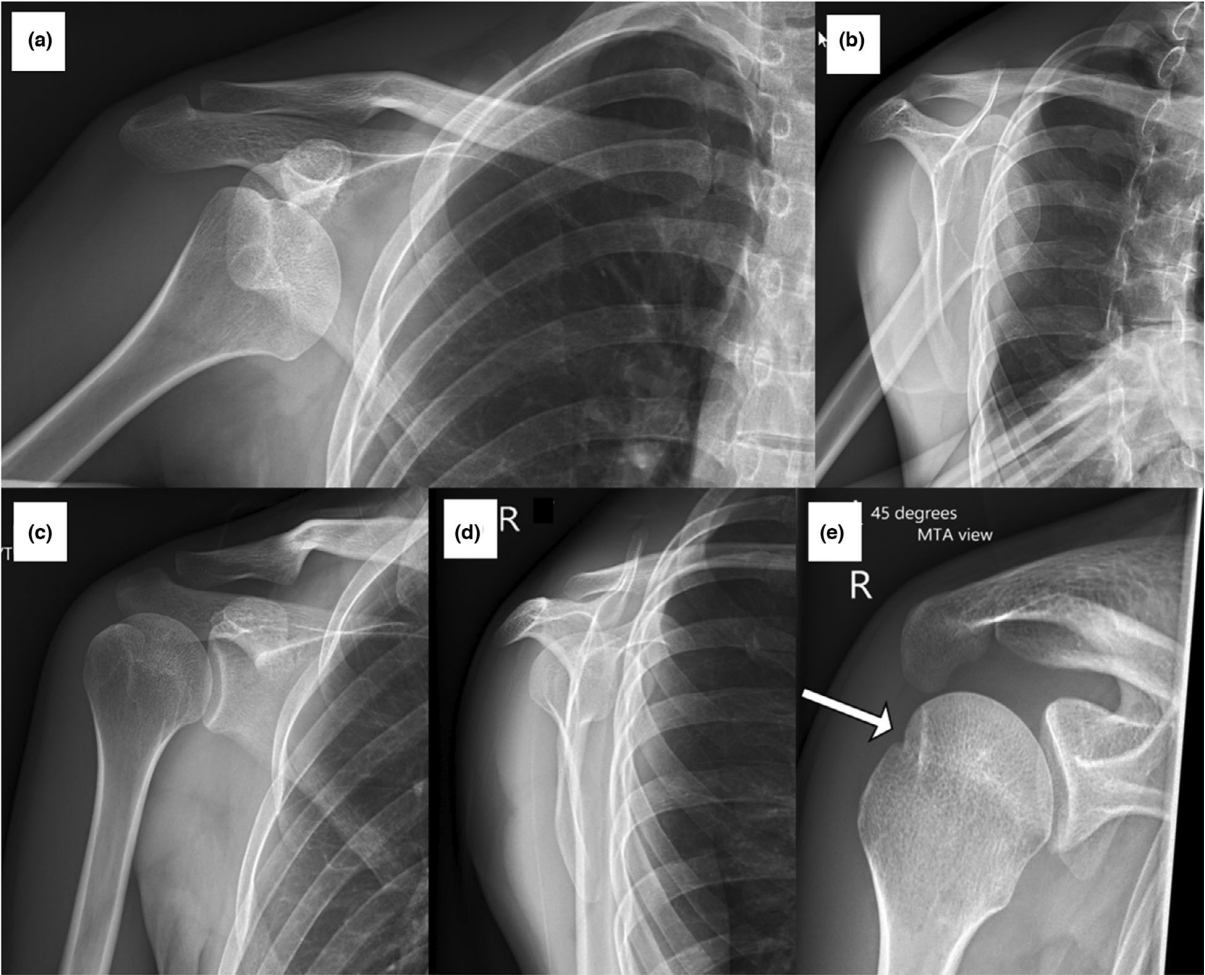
The patient presented for query dislocation. Radiographs were taken pre (A,B) and post (C–E) shoulder reduction. AP (A) and lateral scapula (B) shoulder projections both show anterior dislocation. The post‐reduction AP (C) and lateral scapula shoulder (D) radiographs show no evidence of abnormality. Image E is the MTA view, and a Hill‐Sachs lesion is demonstrated (arrow). MTA, Modified trauma axial.
